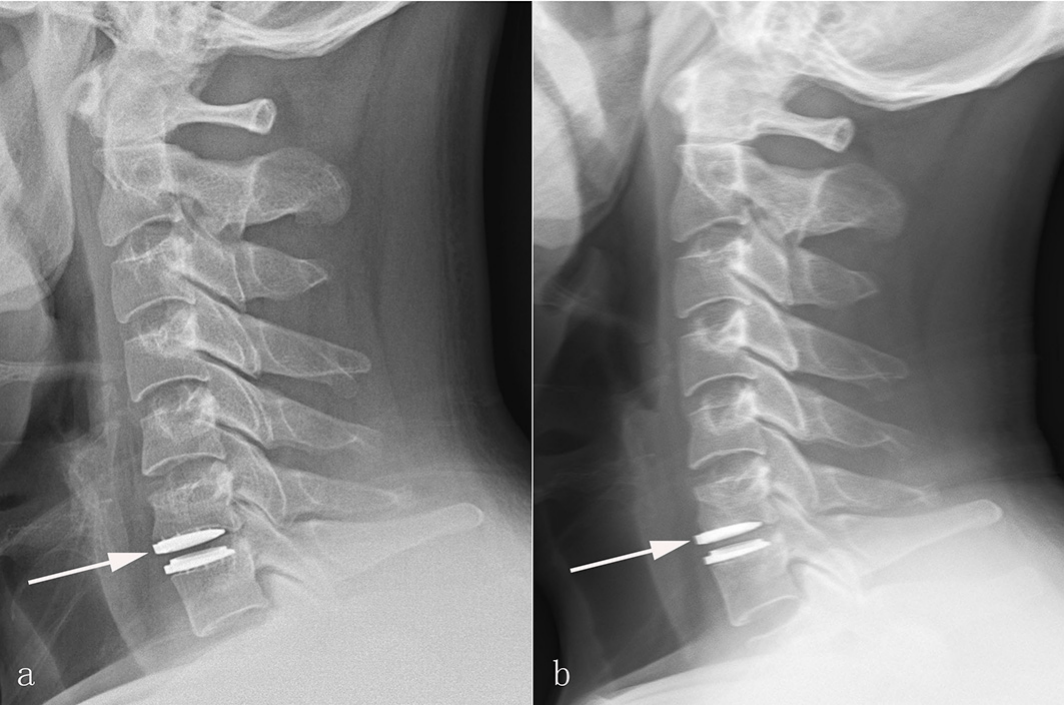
The representive case who suffered migration of Discover prothesis postoperatively. Postoperative 2-day (a), and final follow-up (b) lateral radiographs showing anterior migration of the superior endplate of Discover prosthesis (White arrows).
